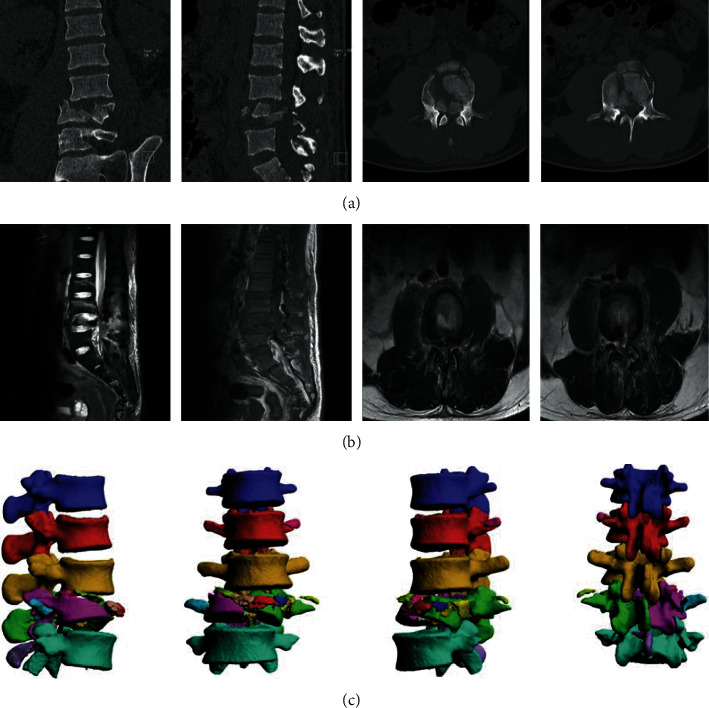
A typical UPA case. A 65-year-old man with LF (L2-L4). (a) CT of lumber presurgery. (b) MRI images of the injured lumbar in the sagittal and the coronal plane. (c) Three dimensional CT images of lumbar.
